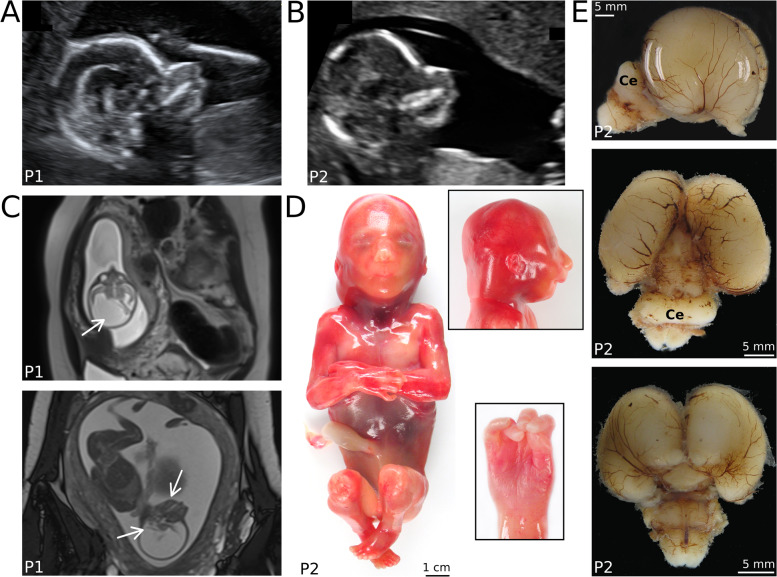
Prenatal clinical and autopsy results. A Prenatal ultrasonography of female fetus P1 at GA 19+1 weeks and B of male fetus P2 at GA 14+3 weeks show comparable micro-/brachycephaly with accentuated flattened short forehead and retromicrognathia in sagittal plane. C T2 axial MRI image at GA 20+6 weeks shows microcephaly and large supratentorial defects of the brain in occipital, parietal, and frontal regions with dilatation and fusion of both side ventricles. Lower panel: T2 TrueFISP sagittal image (GA 20+6 weeks) shows severe cerebellar hypoplasia as well as retrognathia. D Postmortem examination reveals the male fetus with appropriate development, arthrogryposis with camptodactyly (shown in detail), severe micro- and brachycephaly, short receding forehead, and retromicrognathia (shown in detail). E Brain in lateral, occipital, and frontal view presenting micrencephaly, especially affecting frontal lobes and moderate cerebellar hypoplasia (Ce).
